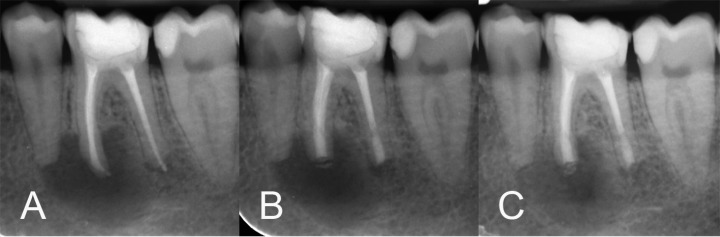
A)* Diagnostic preoperative radiography of the mandibular left first molar exhibits a large radiolucency around the mesial root and a small one around the distal root; *B)* Immediate postoperative radiograph after retrograde treatment and intentional replantation; *C) *2-month follow-up radiograph showing remarkable bone healing in the periapical region
